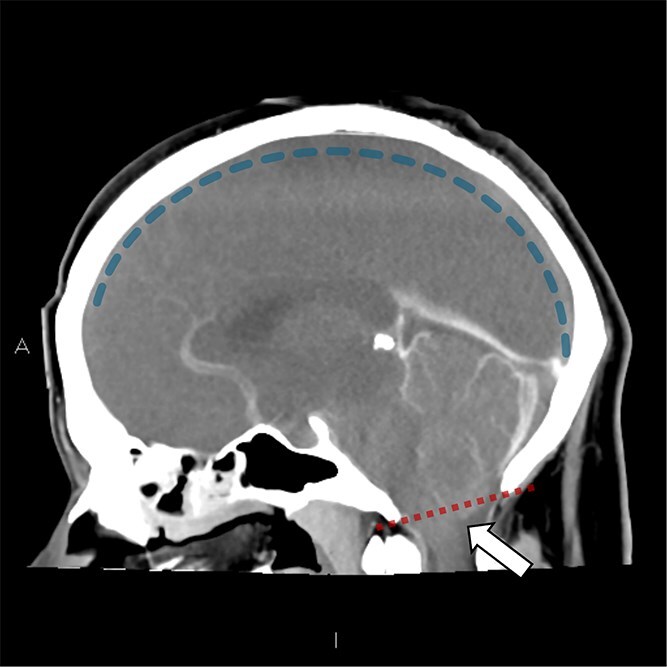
Sagittal slice CT Venogram: Gross cerebral edema with no contrast flow seen within the superior sagittal sinus (blue dashed line) and venous collaterals with early tonsillar herniation (white arrow) indicated by displacement of cerebellum beyond the McRae’s line (radiographic line in red connecting the anterior and posterior margins of the foramen magnum).
